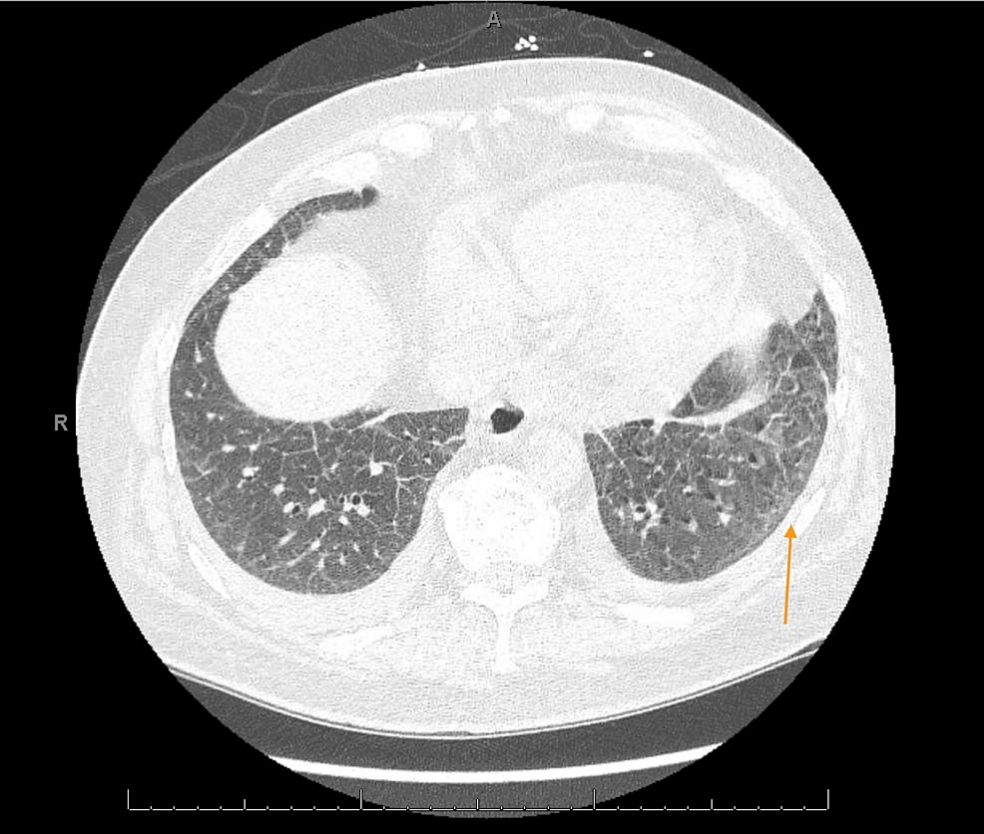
High Resolution CT Chest with Mild Ground Glass Opacities, Left Middle Lobe
